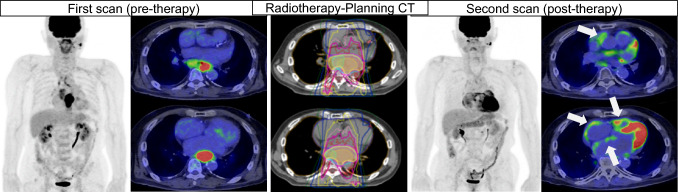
FDG-PET/CT scan before chemo-radiotherapy (left), radiotherapy planning CT (middle), and FDG-PET/CT scan after chemo-radiotherapy (right) in a patient with esophageal cancer with lymph node involvement. High FDG uptake in mid-esophagus and surrounding lymph nodes is noted before the treatment, which disappears after the treatment. New FDG uptakes are observed in the right ventricle and atrium suggesting radiation-induced myocarditis and increased afterload after the treatment. (white arrows)
